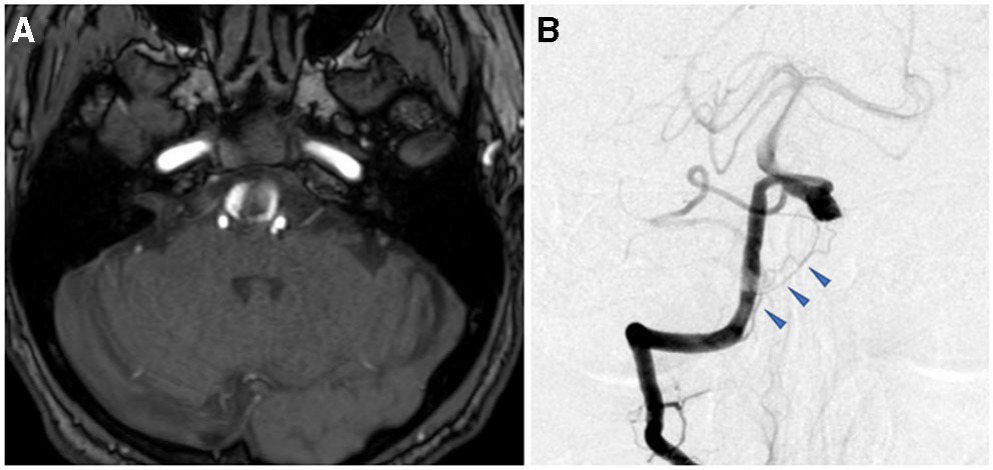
Preoperative image examinations of case 3. (A) Magnetic resonance imaging. (B) Digital subtraction angiography.
